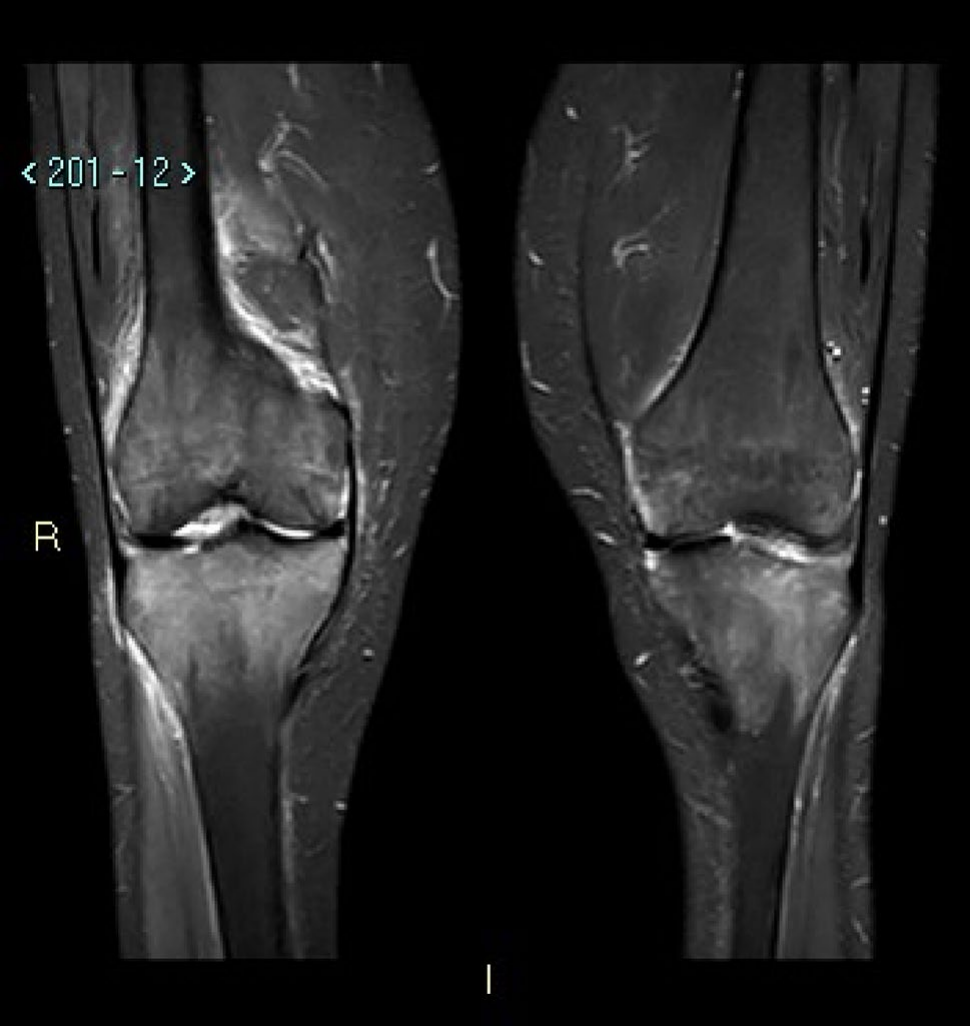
Bilateral knee MRI, six months post-transplant
